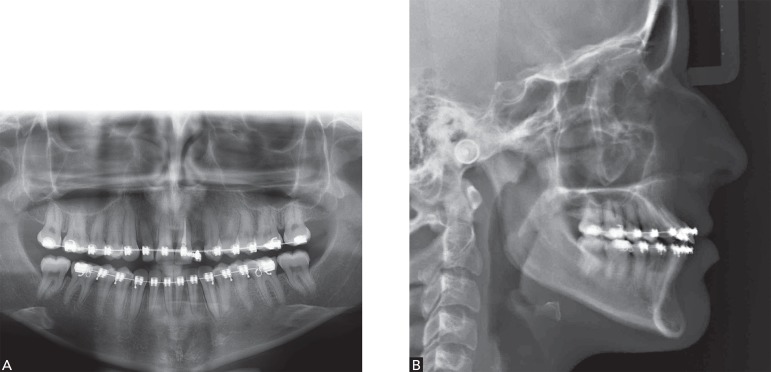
Radiographs at treatment compleA B tion: A) Panoramic;
